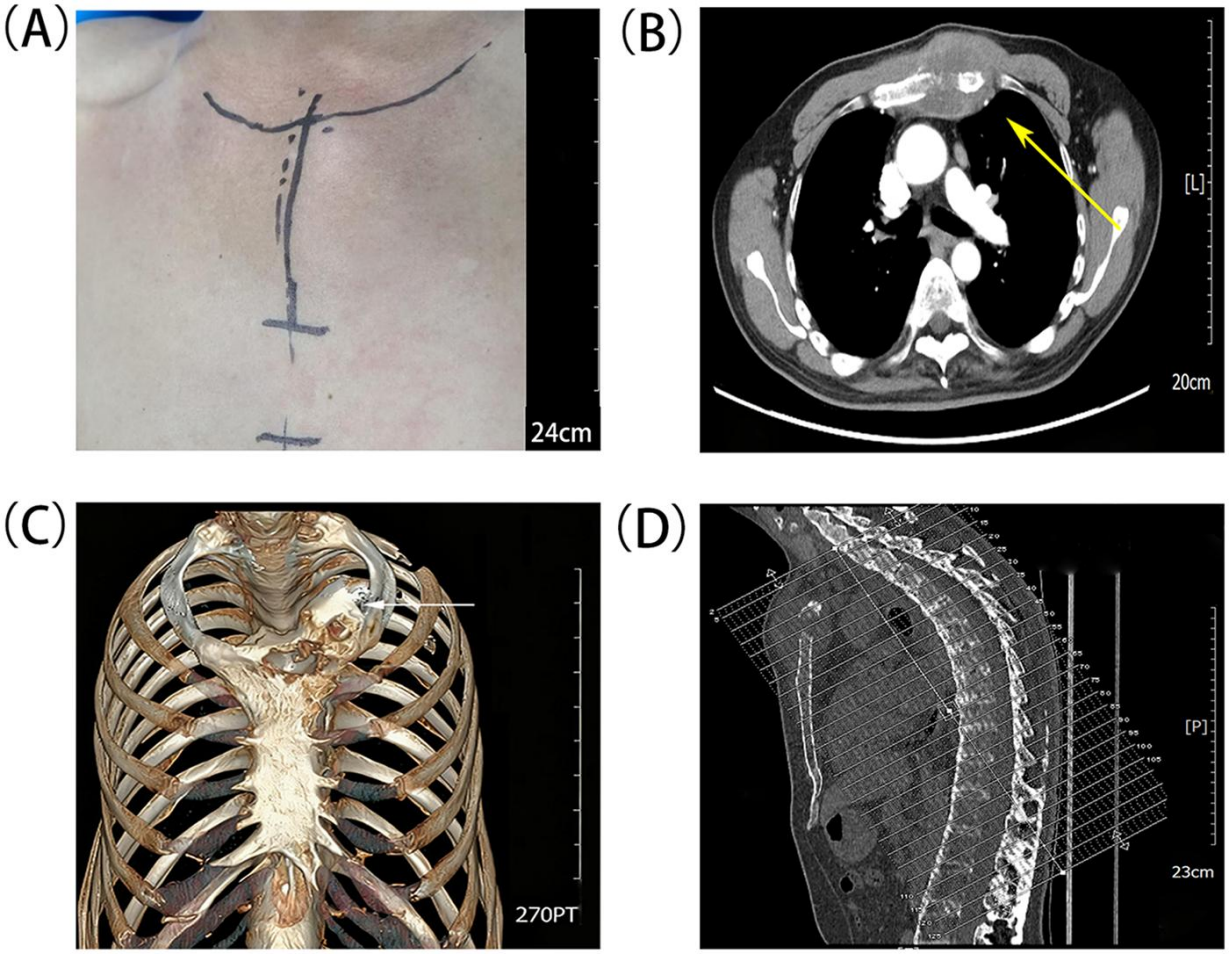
Preoperative computed tomography (CT) images of the sternal malignant tumor. (A) Clinical photograph of the anterior chest wall showing the surface appearance of the tumor lesion prior to surgery, with patient identifying features appropriately protected. (B) Axial contrast-enhanced CT image at the level of maximum tumor thickness showing an expansile destructive lesion in the manubrium sterni with a large soft tissue mass and pathological fracture(yellow arrow). (C) 3D CT reconstruction image demonstrating the extent of tumor invasion, which extended beyond the left sternocostal joint, invading the medial clavicular head and the first and second costal cartilages(white arrow). (D) Sagittal CT image revealing AS, providing an important reference for preoperative surgical risk assessment and surgical planning.
